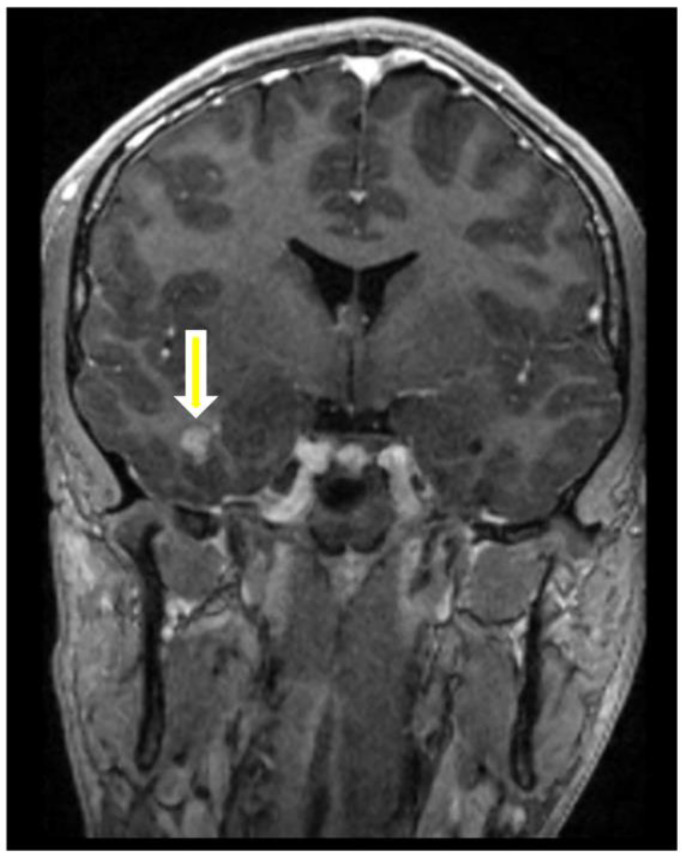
T1-weighted MRI post-contrast MRI revealing a lesion with heterogeneous enhancement in the right medial temporal lobe extending into the cortex (arrow).
